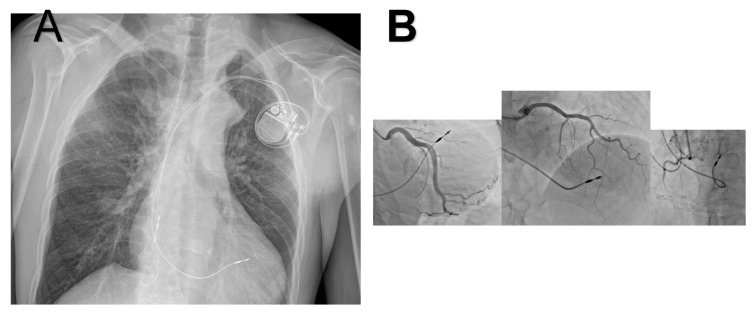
(A) Chest X-ray of thorax and (B) coronary angiography.
